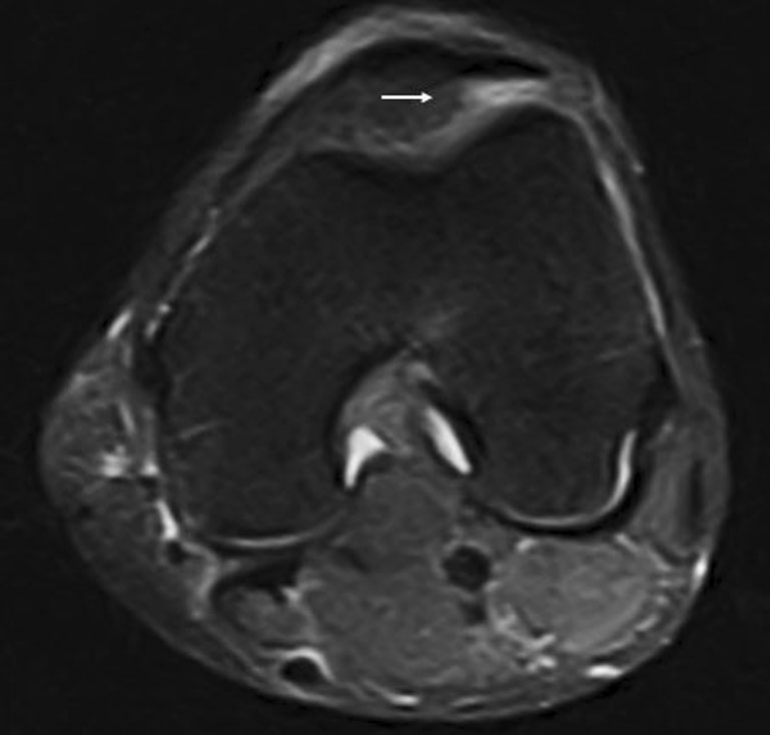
Superolateral HFP impingement. Axial proton density with fat saturation MR image shows oedema in superolateral portion of HFP in a young athlete with persistent knee pain in superolateral portion of the knee
